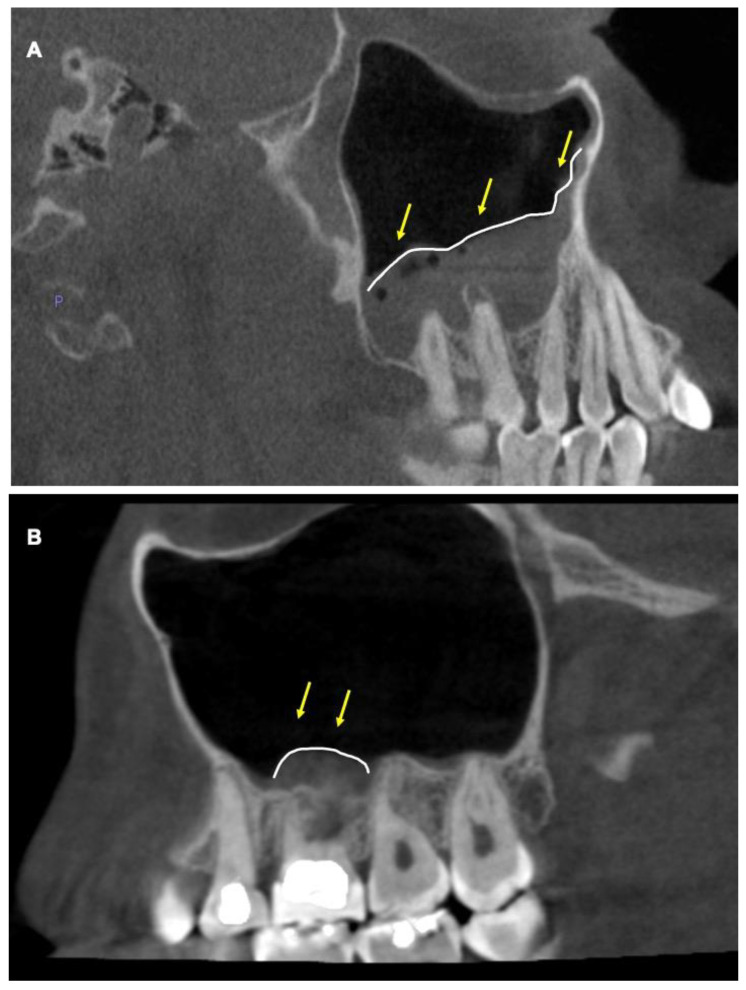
Cone-beam CT images showing mucosal thickening in maxillary sinus. (A) Sagittal view showing generalized mucosal thickening of the left maxillary sinus that extends beyond the maxillary molar area. Yellow arrows pointing to the area. (B) Sagittal view of localized mucosal thickening. Yellow arrows showing mucosal thickening of the left maxillary sinus localized to the maxillary molar area.
